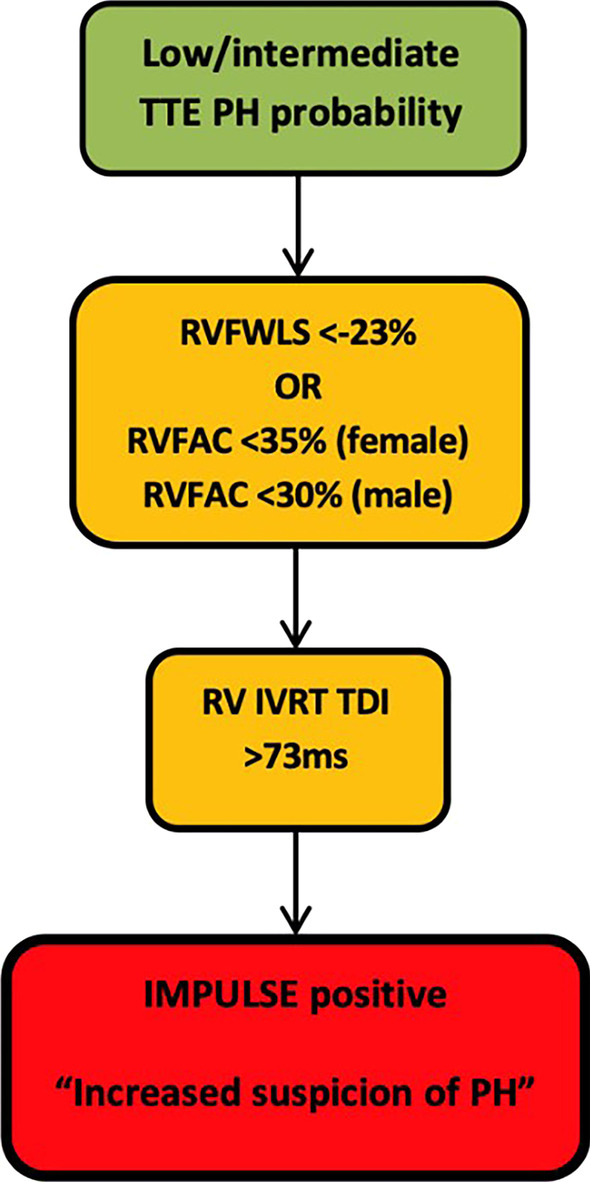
Proposed additional algorithm for those with low or intermediate TTE probability to help identify those with PH and low TTE probability. RVFAC normal cut-off values taken from (Harkness et al., 2020). RVFWLS (right ventricular free wall longitudinal strain); RVFAC (right ventricular fractional area change); IVRT TDI (isovolumetric relaxation time by tissue Doppler imaging); IMPULSE (improving pulmonary hypertension screening by echocardiography)
